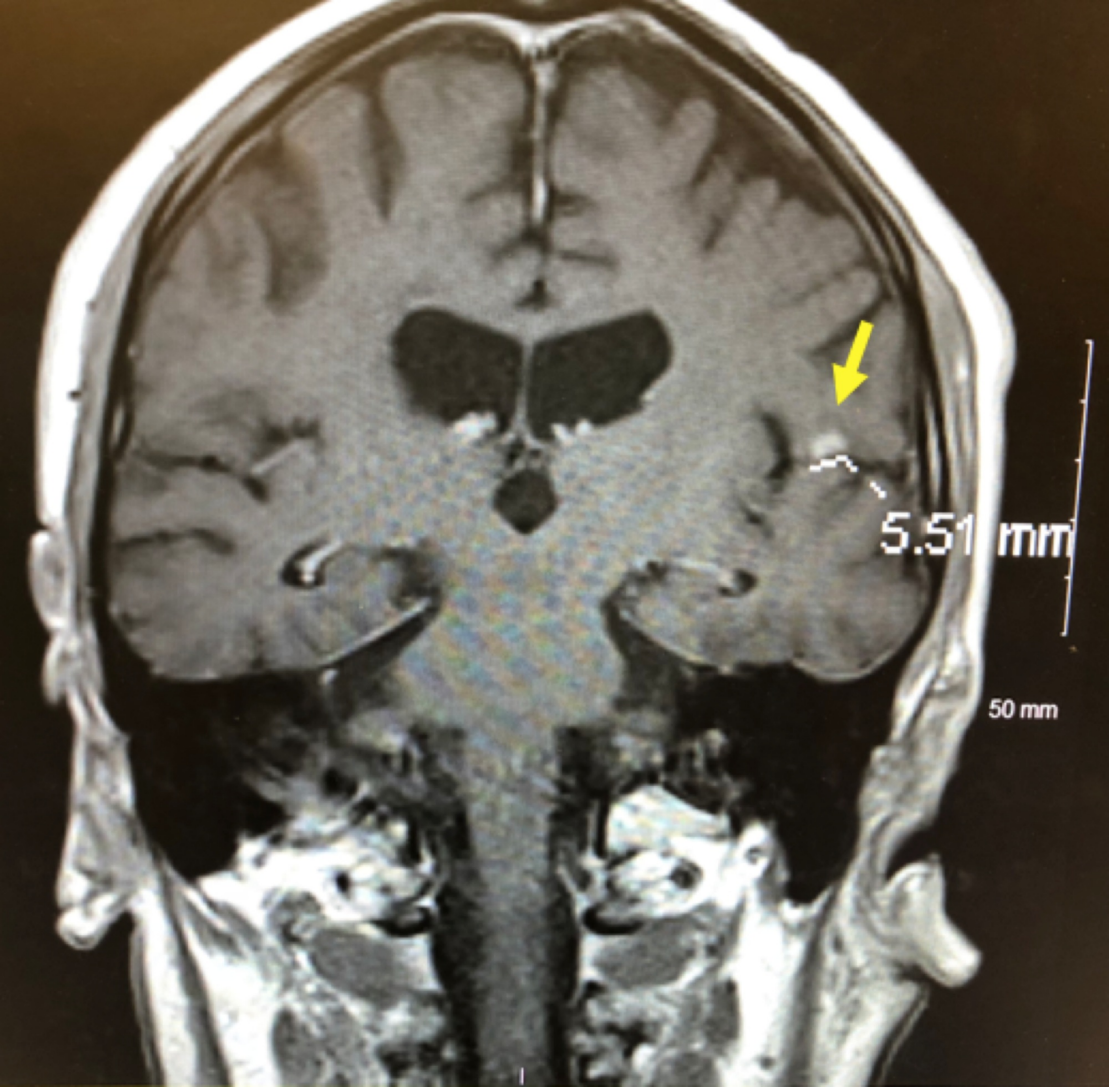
MRI brain with and without contrast demonstrating a 5.51 mm enhancing lesion in the left temporal operculum MRI: magnetic resonance imaging
